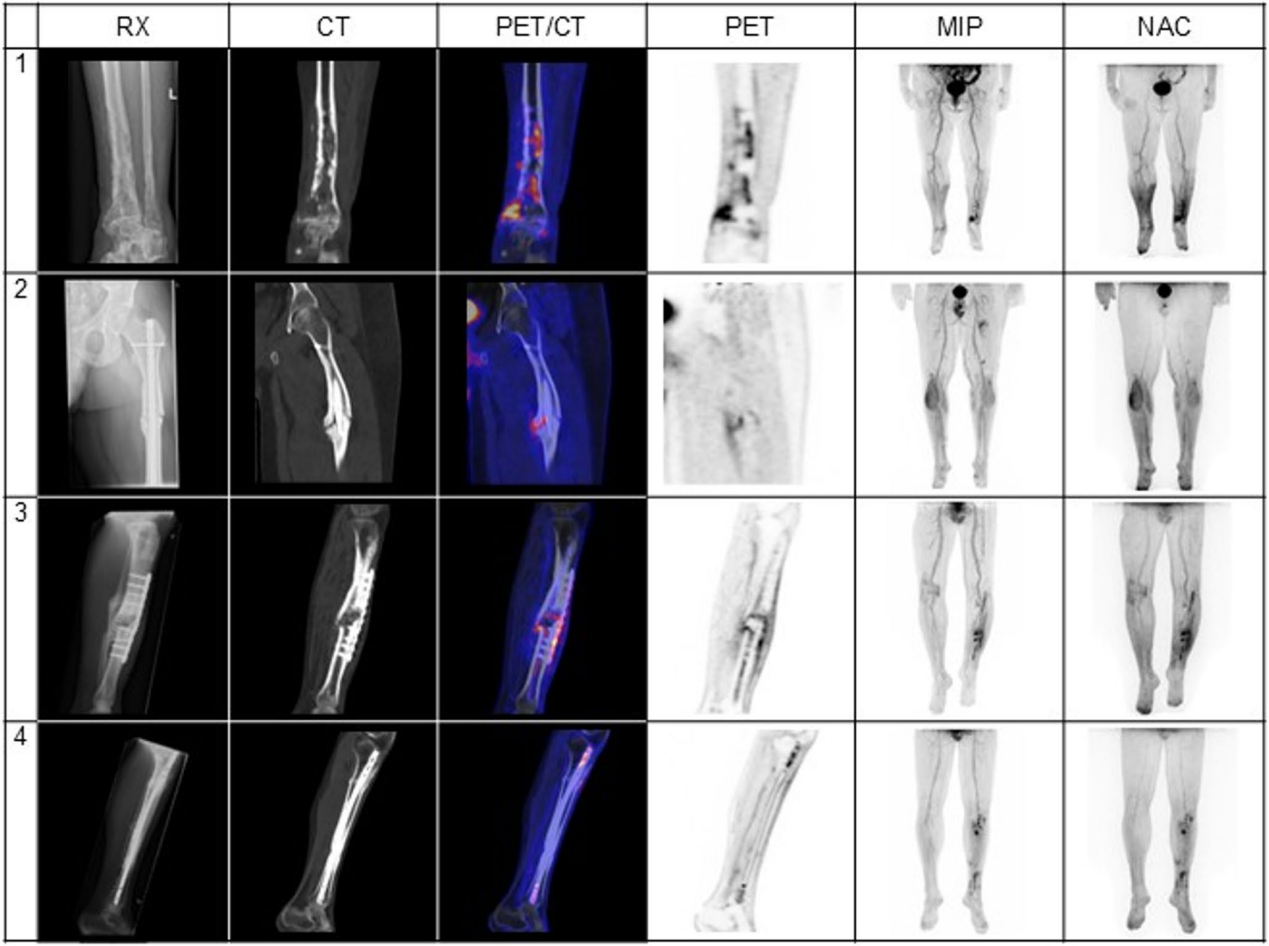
Assessment of standard [^18^F]FDG PET/CT imaging in patients with suspected fracture-related infections. Patient 1 (true positive scan): a confirmed infected non-union of the medial malleolus was classified as true positive as the scan shows intense focal [^18^F]FDG uptake with an SUV_peak_ of 6.65 around the medial malleolus of the left ankle, which persists at the NAC images. Patient 2 (true negative scan): inflammation in a femoral non-union shows a more focal pattern around the fracture site with grade 4, with an SUV_peak_ of 3.31. The [^18^F]FDG uptake at the suspected infection site is not persistent on the NAC image, classifying the scan as true negative. Patient 3 (false positive scan): a tibial non-union shows high focal and heterogeneous uptake at the fracture site and osteosynthesis material with an SUV_peak_ of 4.75, which is persistent at the NAC image, but no infection could be confirmed based on the criteria of the fracture-related infection consensus definition. Patient 4 (visual assessment: true positive scan, quantification: false negative scan): a tibial non-union shows high focal uptake around the threaded holes of the osteosynthesis material, more pronouncedly proximal with a SUV_peak_ of 3.86, which persist on the NAC images. This patient was correctly classified as infection by visual scan analysis, but falsely classified as negative by quantification (SUV_peak_ < 4.40). Abbreviations: RX = radiograph, CT = computed tomography, PET = positron emission tomography, MIP = maximum intensity projection, NAC = non-attenuated corrected, SUV = standardized uptake value
